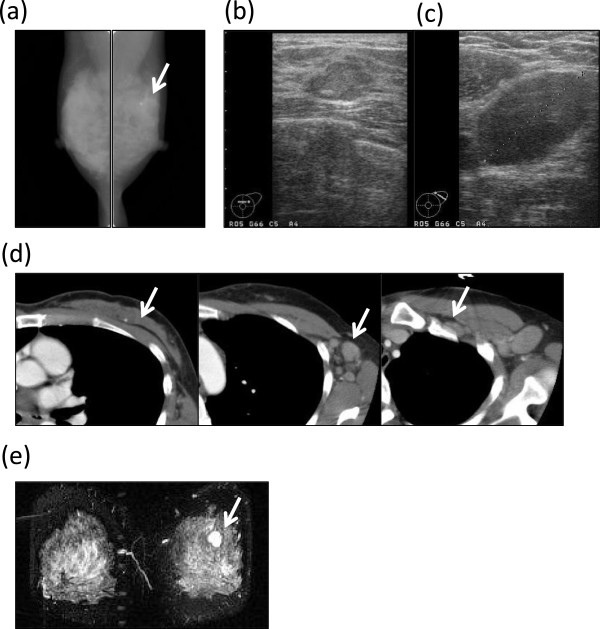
Imaging findings. (a) Mammography showed focal asymmetrical density in the left upper area of the left mediolateral oblique position (arrow). (b) Ultrasonography confirmed the presence of a 21 mm in diameter, lobulated, low- to iso-echoic area with a high echo spot, the border of which was unclear. (c) The ipsilateral axillary lymph nodes were swollen, the largest of which was 32 mm in diameter. (d) A CT scan revealed a low enhanced mass with calcification in the middle of upper area of the left breast (left), and the ipsilateral axillary (middle) and subclavicular lymph nodes (right) were swollen. (e) MRI revealed a lobulated mass with high intensity in the middle of upper area of the left breast.; CT, computed tomography; MRI, magnetic resonance imaging.
